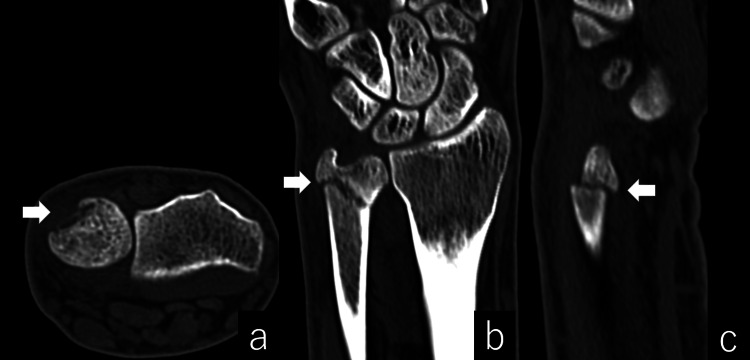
Computed tomography scan. Axial (a), coronal (b), and sagittal (c) views showed a 1-mm step-off on the dorsal side at the groove.
